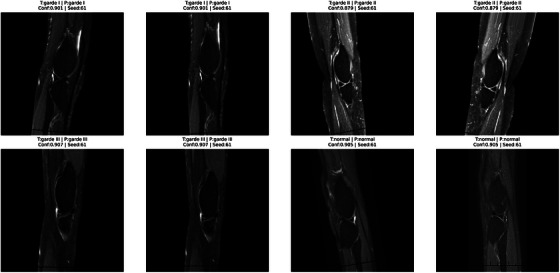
Representative correctly classified MRI samples from the test set across all classes.
